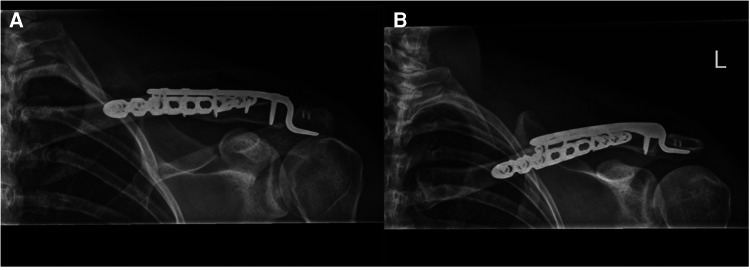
Radiographs demonstrating fixation of the fracture and dislocation with a hook plate and anterior clavicle plate.
